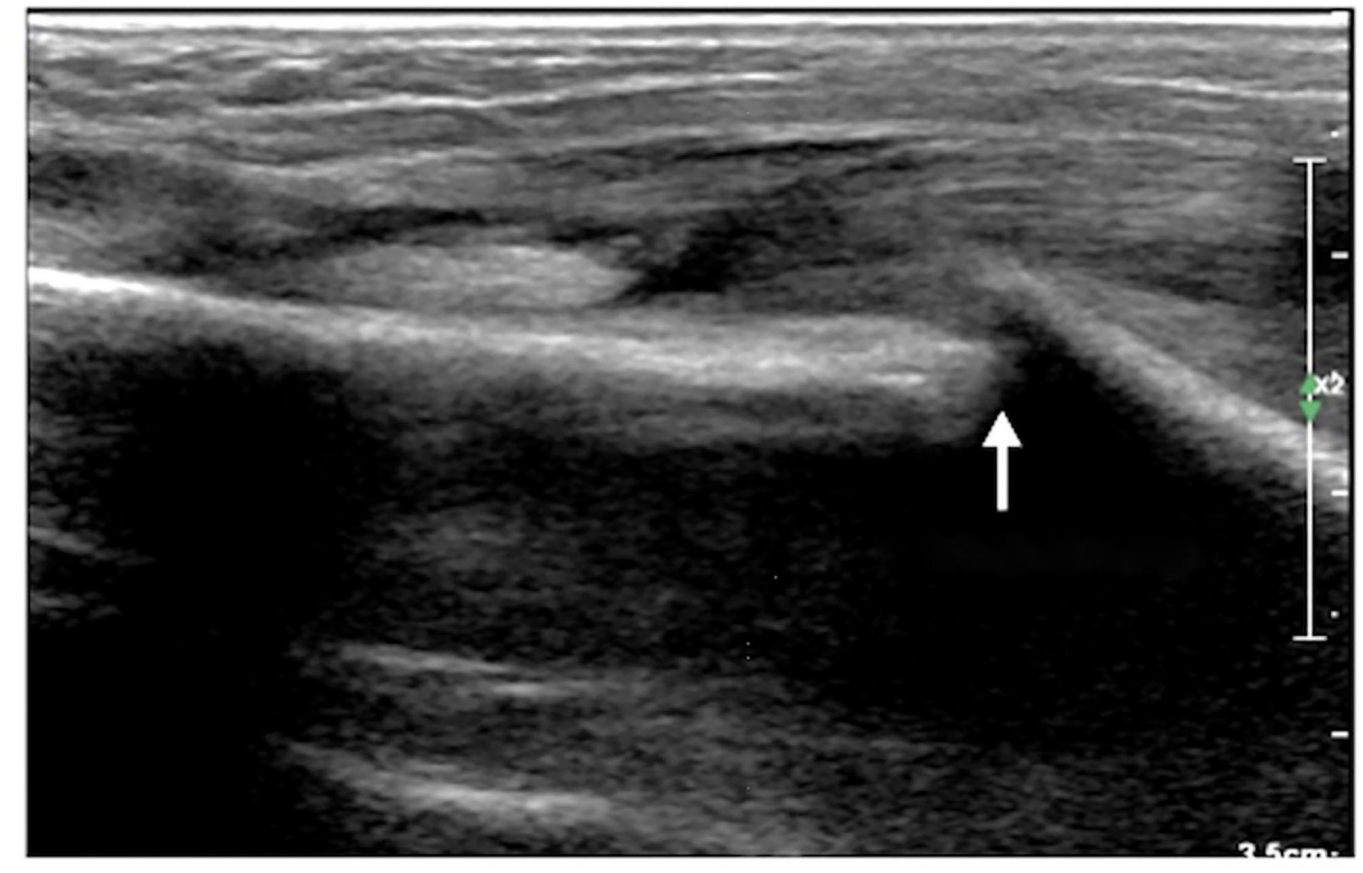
Humeral fracture. The infant was G_2_P_1_ with a gestational age of 39^+2^ weeks, forceps delivery, and birth weight of 4,000 g. The patient was admitted to the hospital 20 h after birth due to a 6-h fever. The diagnosis was intrauterine pneumonia. Physical examination revealed swelling, tenderness, limited mobility, and loss of the primitive reflex of the right upper limb; thus, the fracture was suspected. Ultrasound showed interrupted cortical continuity, visible broken ends, displacement, separation, and angulation of the right humerus.
